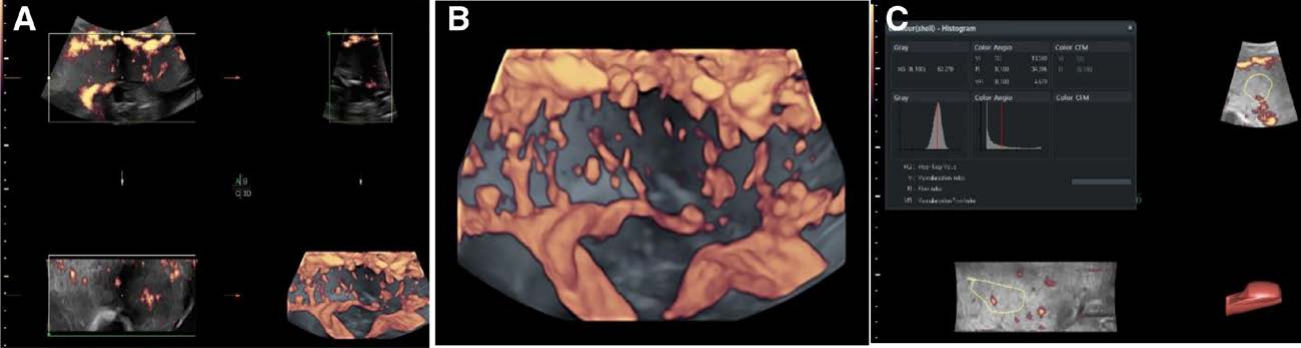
Detection of placental 3-dimensional power Doppler index. (A) Volumetric data acquisition; (B) Three-dimensional image of placental blood vessels; (C) VOCAL software quantitative analysis. VOCAL = virtual organ computer-aided analysis.
